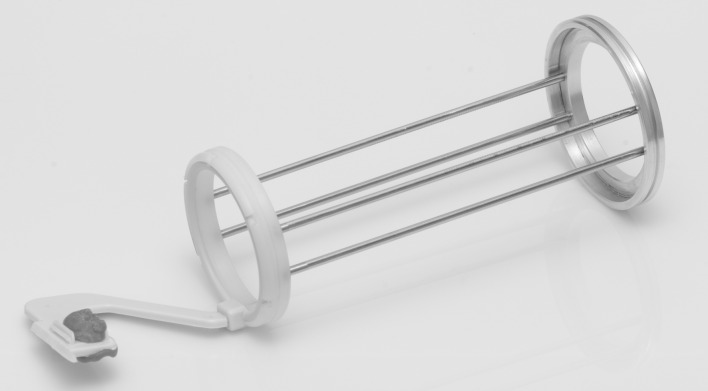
Standardizing device of each patient attached to an individual radiographic positioner with occlusal record in acrylic resin.
